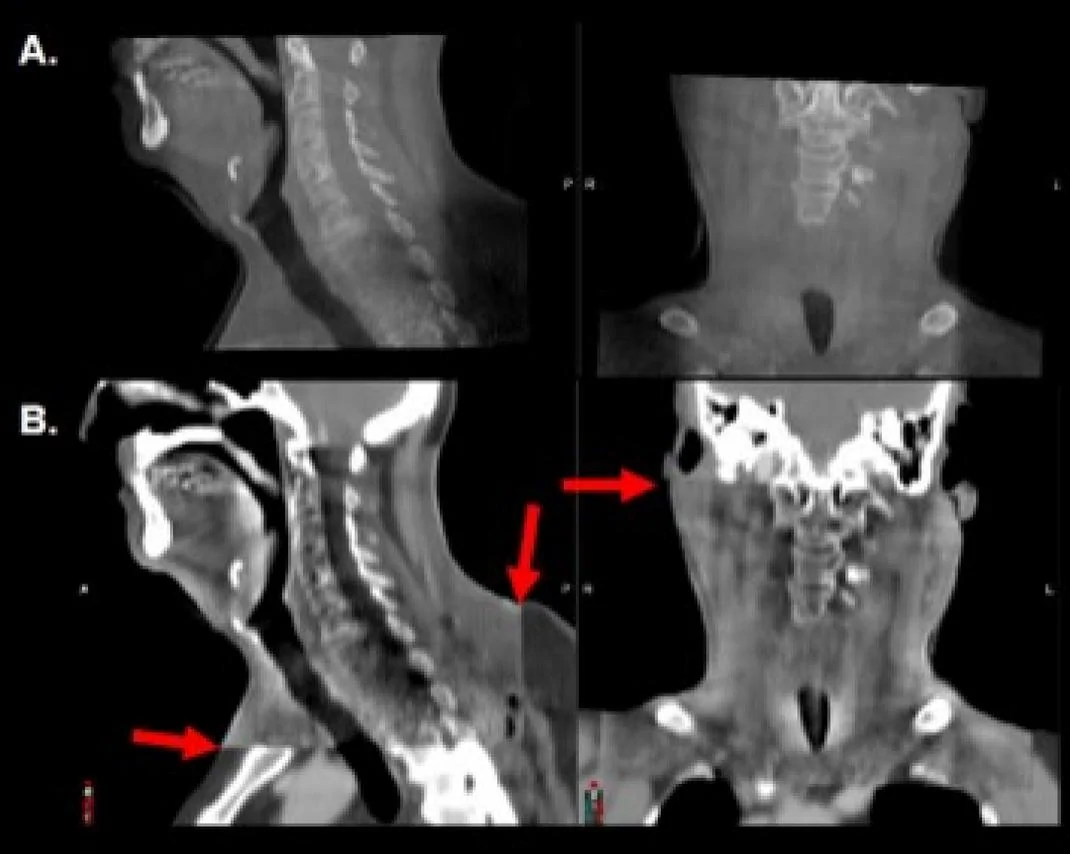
Example CBCT images before (A) and after (B) processing for dose recalculation. Processing includes merging with the original planning CT outside the CBCT field of view (red arrows) and Hounsfield unit intensity normalization, producing an enhanced CBCT suitable for Monte Carlo dose calculation. (For interpretation of the references to colour in this figure legend, the reader is referred to the web version of this article.)
